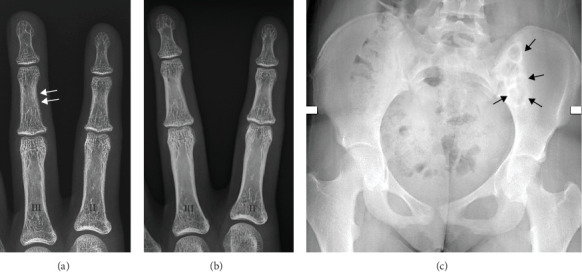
Bone changes before (A) and after treatment (B). Images were cropped and magnified from anteroposterior radiographs of the hands. II: index finger. III: third finger. (A) Before treatment, bone density is decreased, with a lace-like appearance of the cortex and subperiosteal bone resorption (arrows), signs of hyperparathyroidism. (B) After surgery and initiation of medical treatment, bone reconstruction with a normal appearance of bone density and cortex. (C) Anteroposterior radiograph of the pelvis showing a well circumscribed lytic lesion of the left iliac bone with sclerotic borders (arrows). In this context, it represents a brown tumor.
